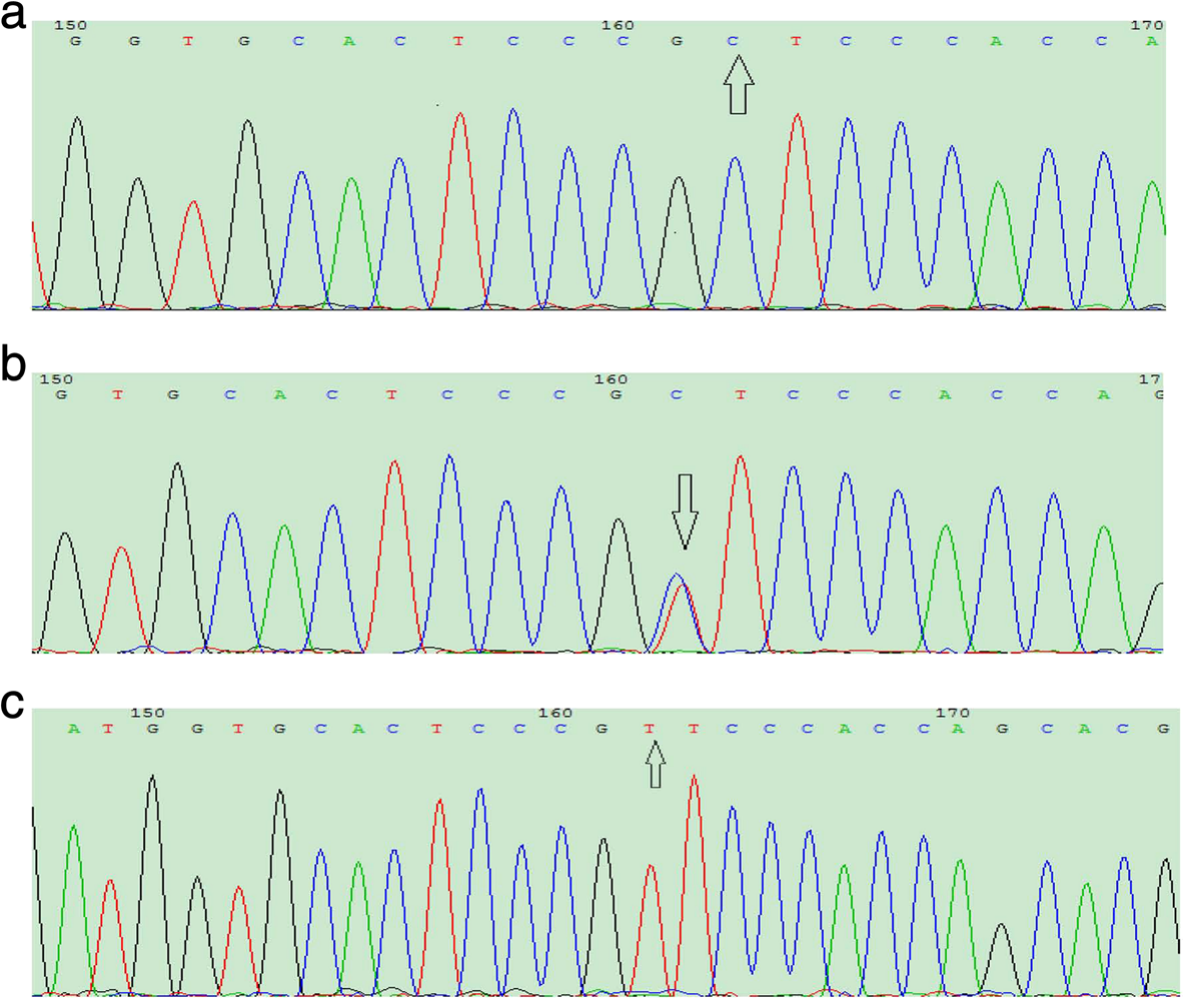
The sequence of CC, CT, and TT genotype, the arrow showed the mutation (a: CC, b: CT, c: TT).
